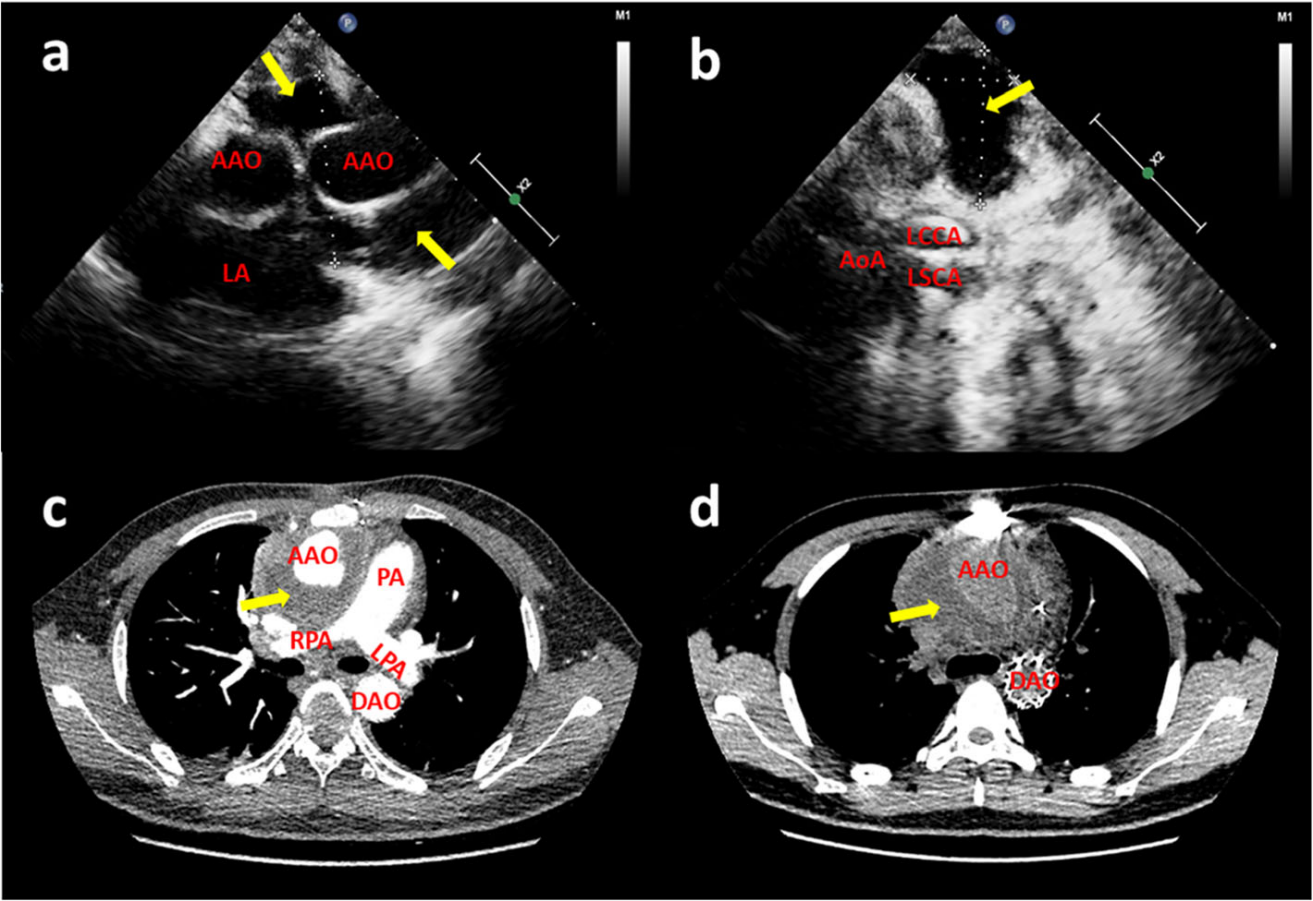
(a) Transthoracic echocardiography revealed liquid dark area and a little thrombus around the artificial blood vessels (yellow arrow); (b) The suprasternal view revealed a liquid area of the abscess (yellow arrow); CT angiography scan (c and d) showed perigraft liquid with low density around the artificial blood vessels (yellow arrow); Intraoperative photographs showed (AAO: ascending aorta; AoA:aortic arch; DAO: descending aorta; LA: left atrium; LCCA: left common carotid artery; LPA: pulmonary artery; LSCA: left subclavian artery; PA: pulmonary artery; RPA: right pulmonary artery)
